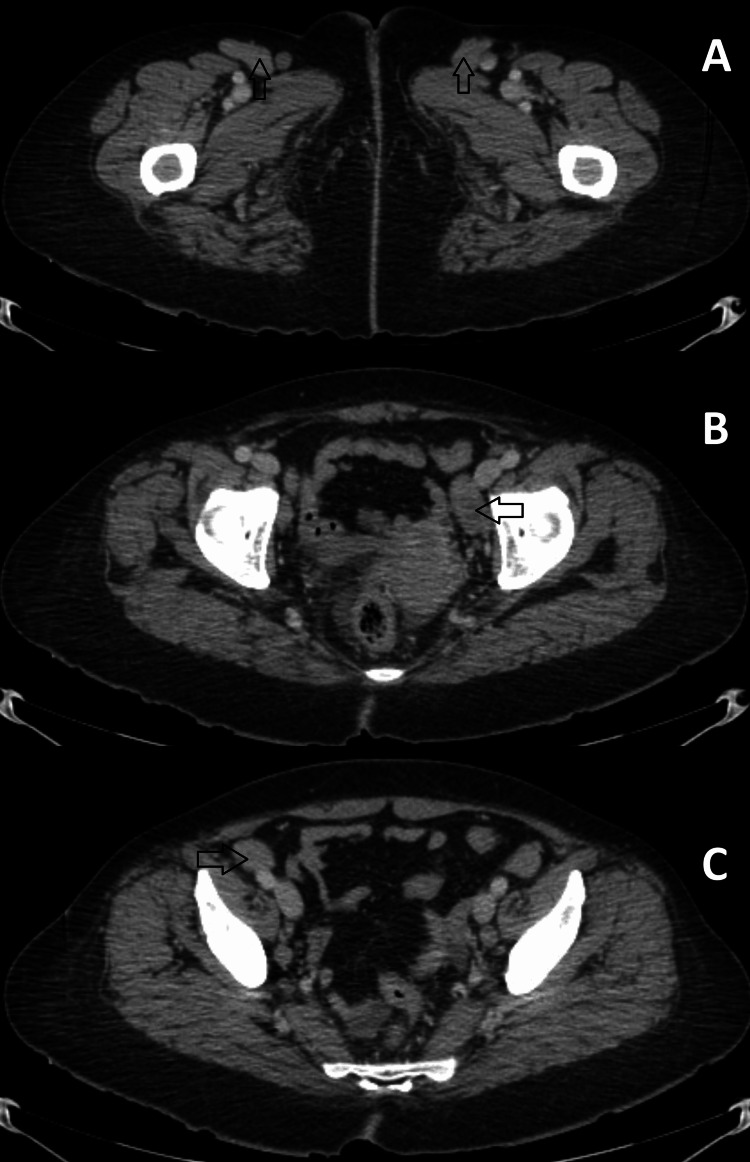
Contrast-enhanced abdominal CT scan in venous phase showing multiple adenopathies (A) Right (3.8 x 1.3 cm) and left (2.9 x 1.4 cm) inguinal adenopathies (arrows); (B) Left external iliac adenopathy (2.9x 1.4 cm) (arrow); (C) Right external iliac adenopathy (2.6 x 1.8 cm) (arrow).
